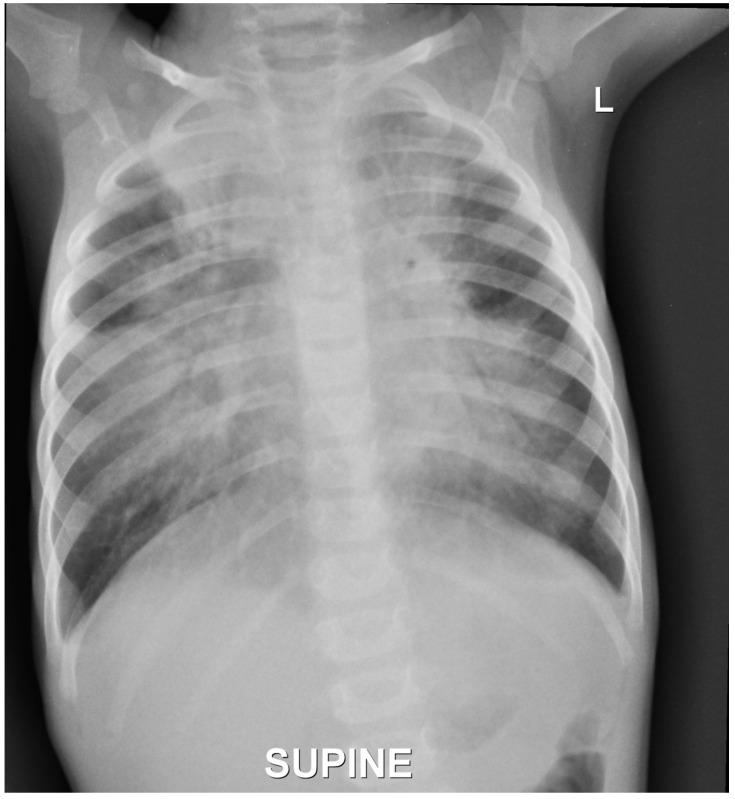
Chest X-ray showing extensive bronchopneumonia.
